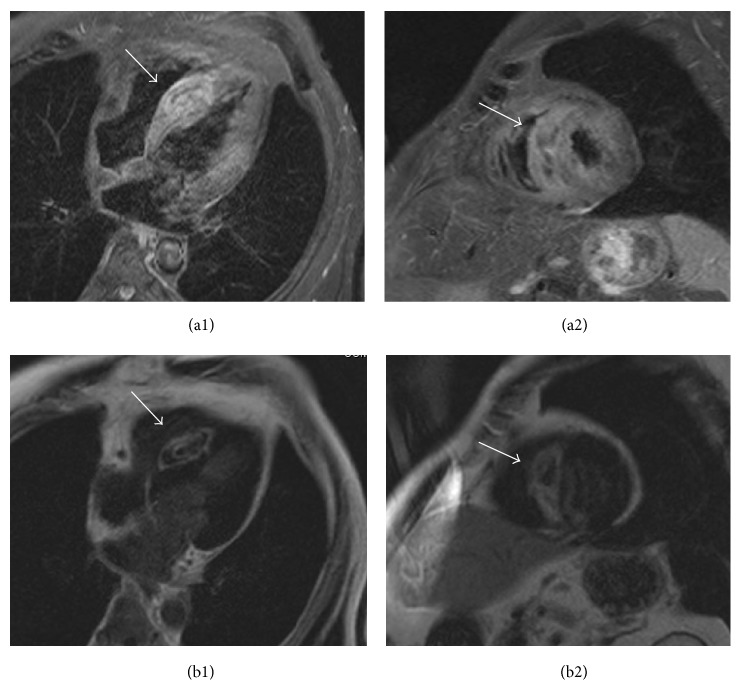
Interventricular septum with hematoma accompanied with microvascular obstruction by cardiac magnetic resonance (CMR) imaging performed 6 days after the index PCI. (a) T2-weighted image. (b) Late gadolinium enhancement (LGE) image.
